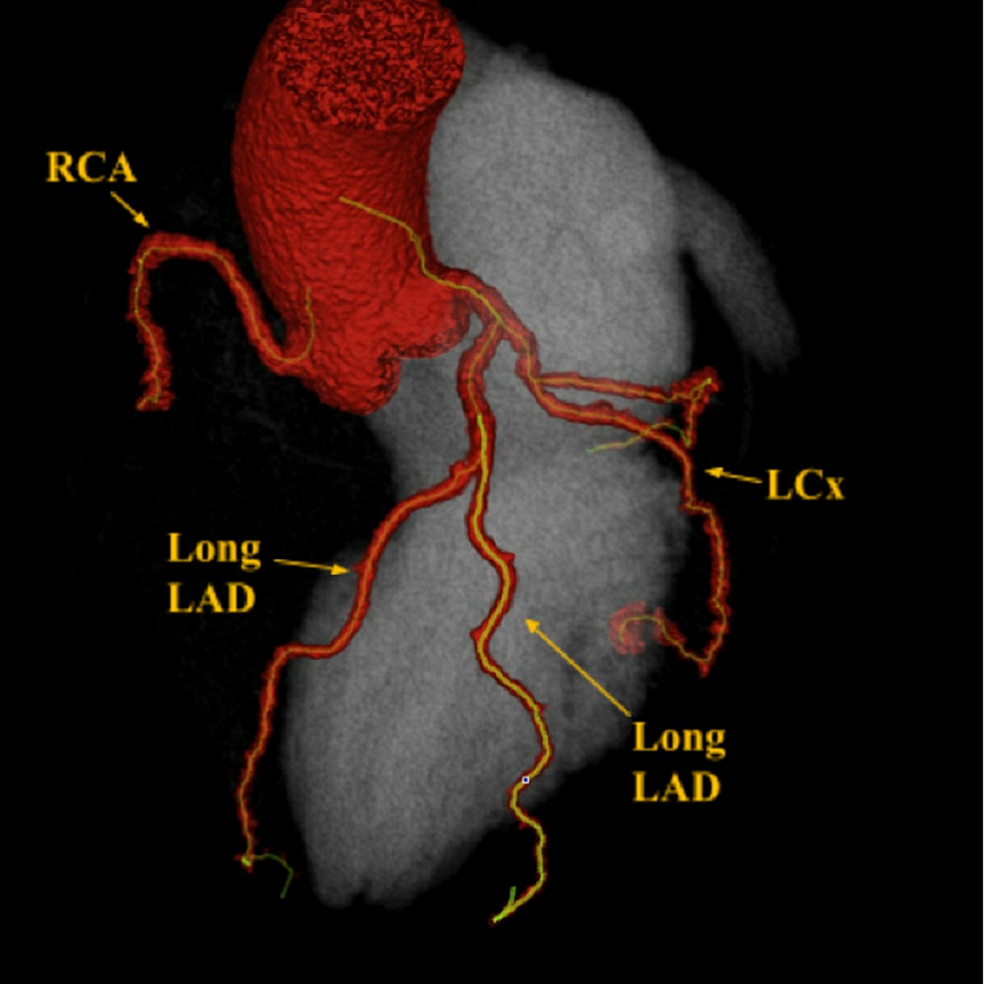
CCT showing two long LADs CCT: Coronary computed tomography; LAD: Left anterior descending artery.
